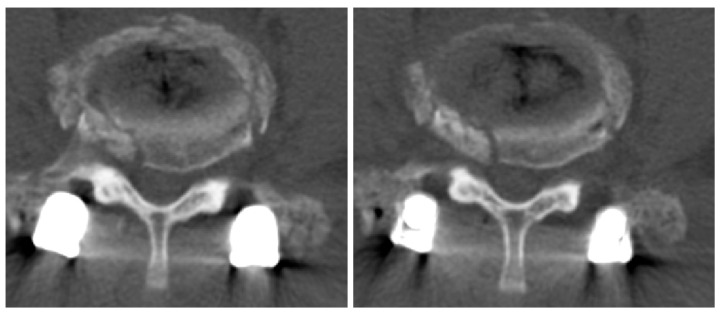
CT image 1 month after first surgery showing post-traumatic stenosis on the right side at the L4/L5 level.
